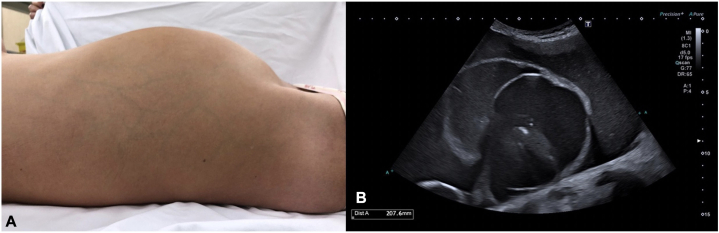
A, Abdomen of the patient when referred to the oncohematology unit. B, Abdominal ultrasound findings with a solid-cystic mass that occupied almost the entire pelvis and extended to the upper abdomen.
